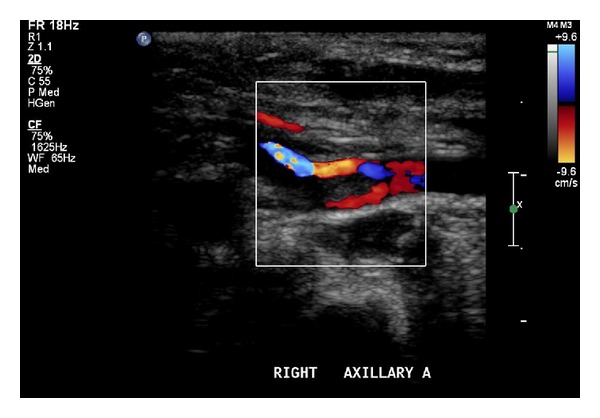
Ultrasound scan of the right axillary artery demonstrating a substantial non-occlusive hypo-echoic thrombus.
